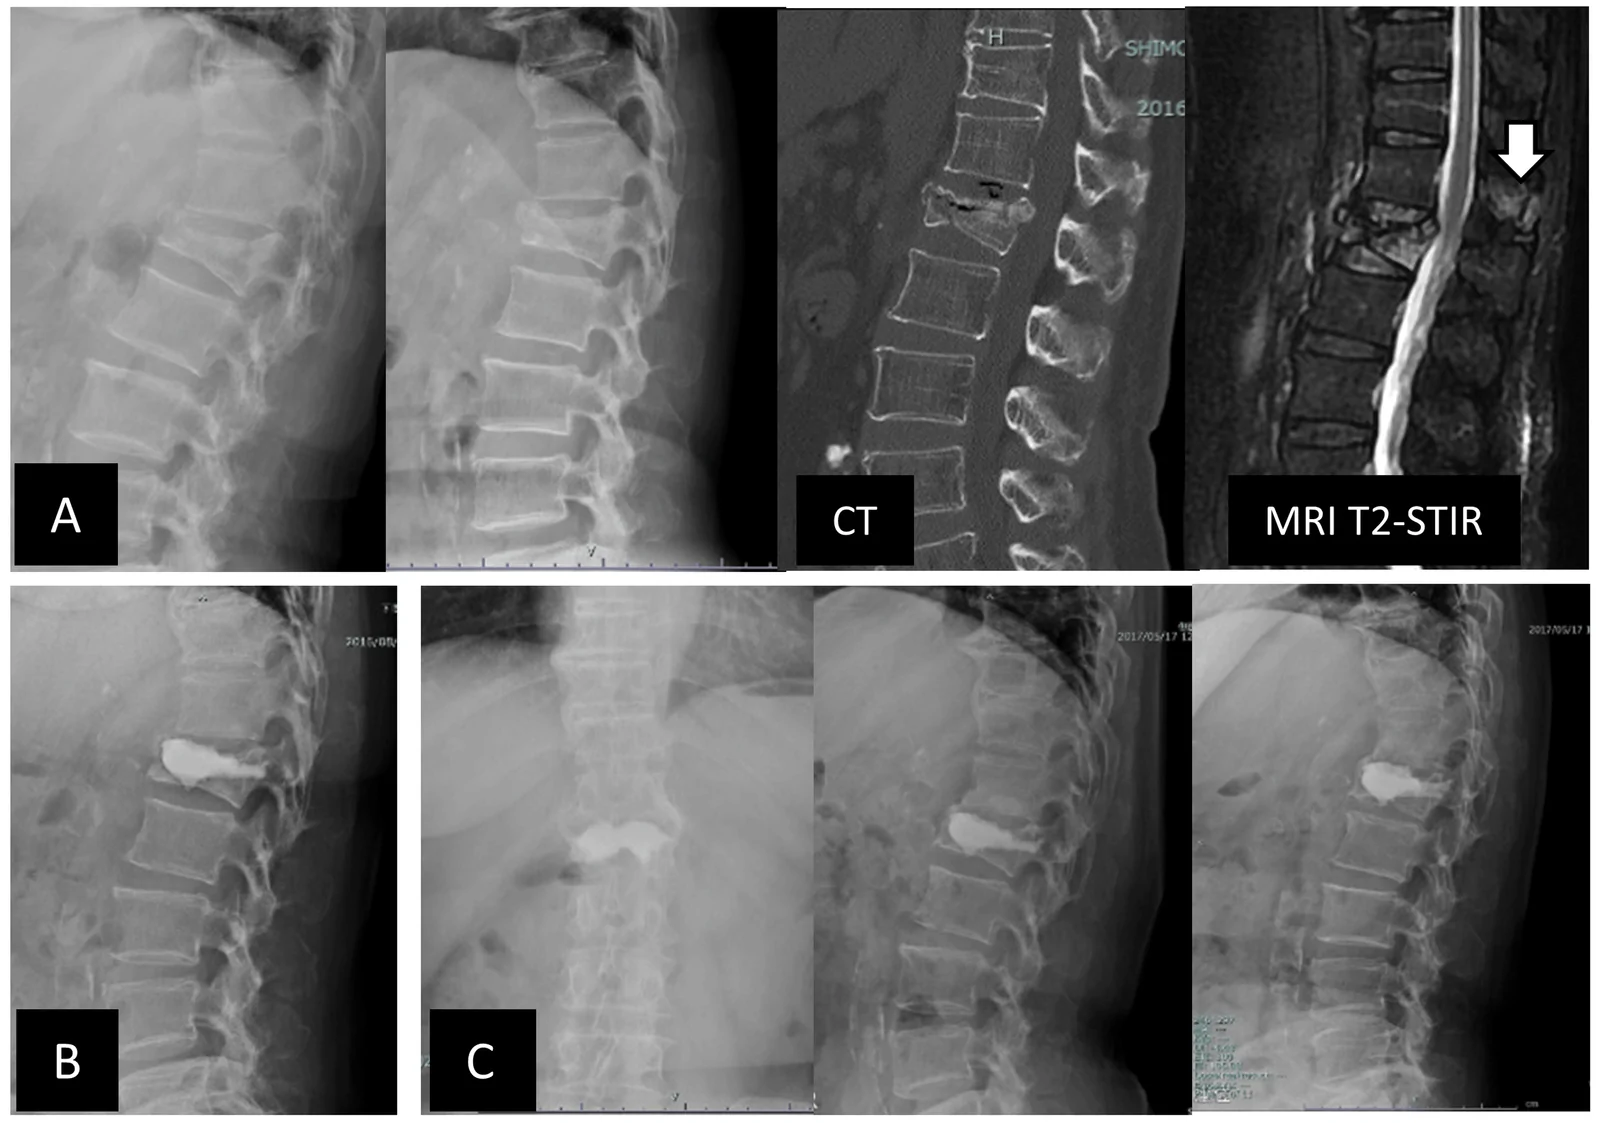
Preoperative and postoperative images of a 74-year-old female with a T12 vertebral fracture A: Preoperative images: from the left - radiographs (supine lateral, seated lateral), computed tomography (CT), magnetic resonance imaging (MRI) (T2-STIR). White arrow indicates the spinous process fracture line; B) One-week postoperative radiograph (lateral view in the lateral decubitus position); C) One year postoperative: from the left - radiographs (anteroposterior, supine lateral, seated lateral).
